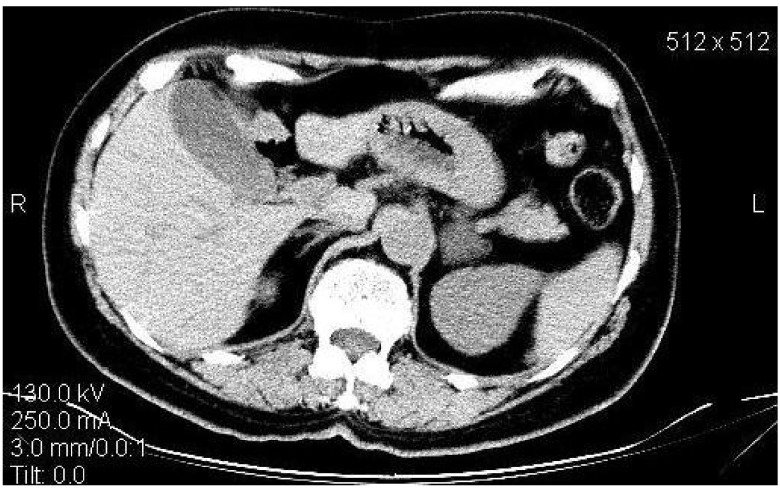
CT scan visualizing 35 mm tumor in the left adrenal gland and a normal right adrenal gland.
